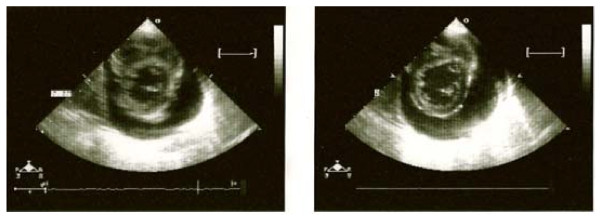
Echocardiogram showing a large anterior and posterior pericardial effusion causing right atrial and ventricular collapse.
